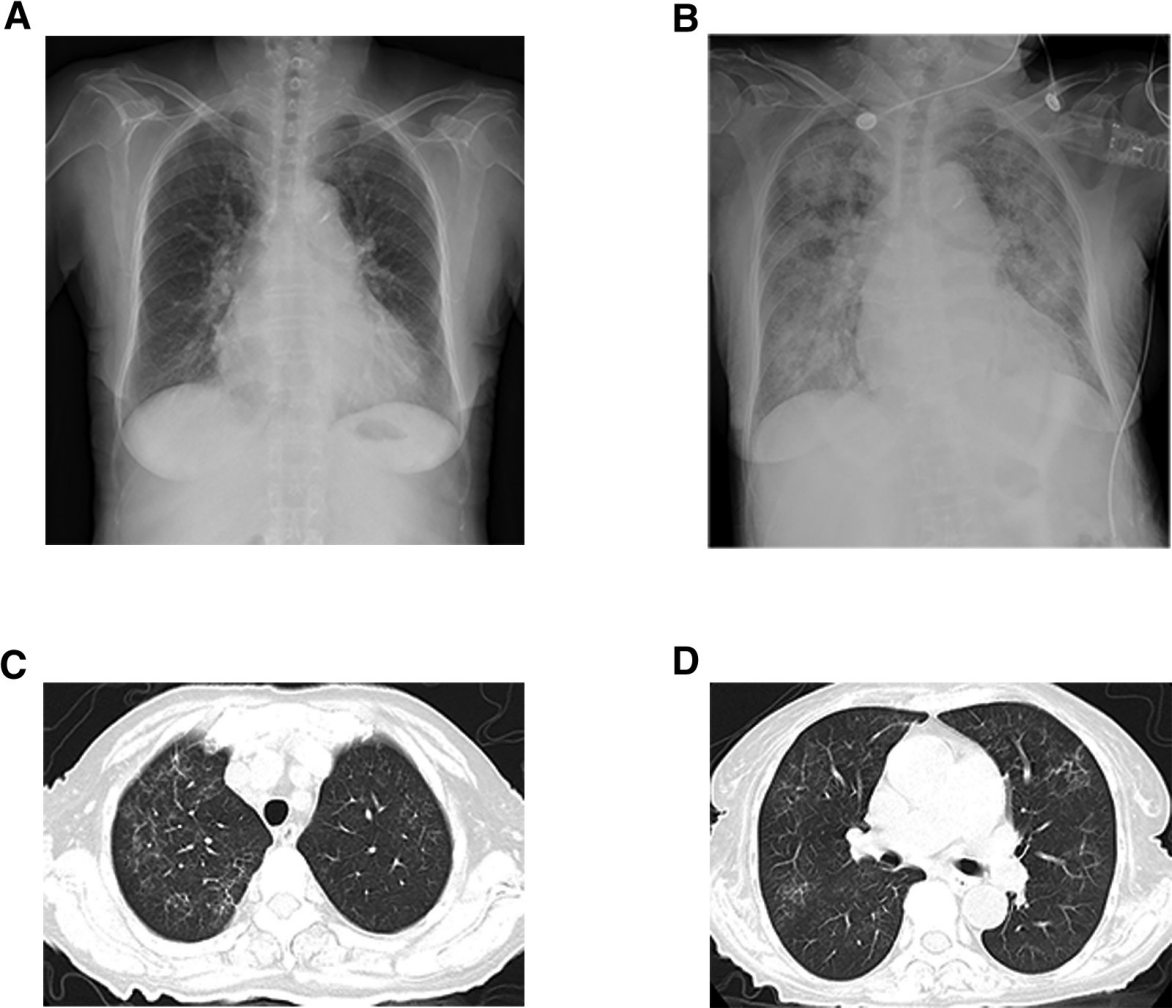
Radiographic and CT scan findings of the patient. (A) X-ray on July 13, 2023. (B) X-ray on July 27, 2023. (C) and (D) Chest CT scan results showing interstitial pneumonia on August 9, 2023.
